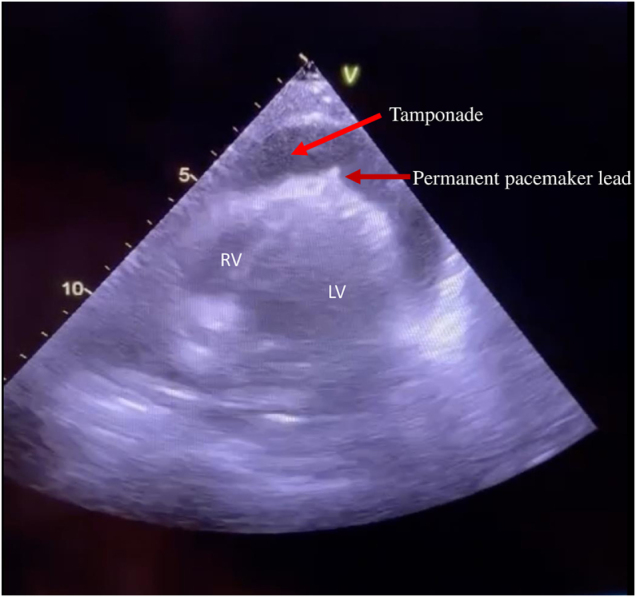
Transthoracic echocardiography showing massive pericardial effusion compressing the right ventricle. The pacemaker lead is seen protruding outside the pericardial sac.
